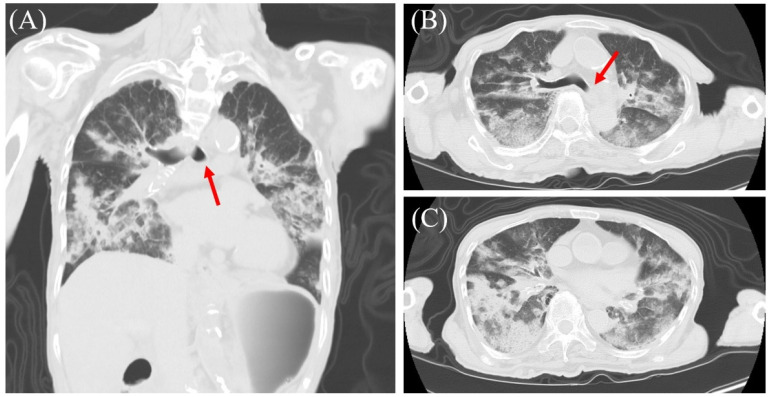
Autopsy imaging results obtained using computed tomography (CT). (A–C) CT scans showing obstruction of the left main bronchus (arrows) and bilateral consolidations consistent with severe pulmonary edema over large areas of both lung fields. The patient was an 83-year-old woman who found collapsed at home by her family. She had a history of diabetes mellitus, dyslipidemia, Alzheimer’s disease, post-operative colon cancer, cataracts, and a rib fracture. She also had a history of rheumatoid arthritis (RA), diagnosed at age 71, for which she was treated with methotrexate at age 78. There was no clinical history of chronic heart failure, valvular heart disease, or chronic obstructive pulmonary disease (COPD). Echocardiography performed 9 years prior showed a preserved ejection fraction (69%) with only mild mitral and tricuspid regurgitation. However, she discontinued her oral medication and stopped attending appointments based on her own judgment, without consulting her doctor. She was transported to our hospital in cardiopulmonary arrest, but resuscitation efforts were unsuccessful, and death was confirmed 1 h and 19 min after her family discovered her. Autopsy imaging and a pathological autopsy were performed at the time of death.
